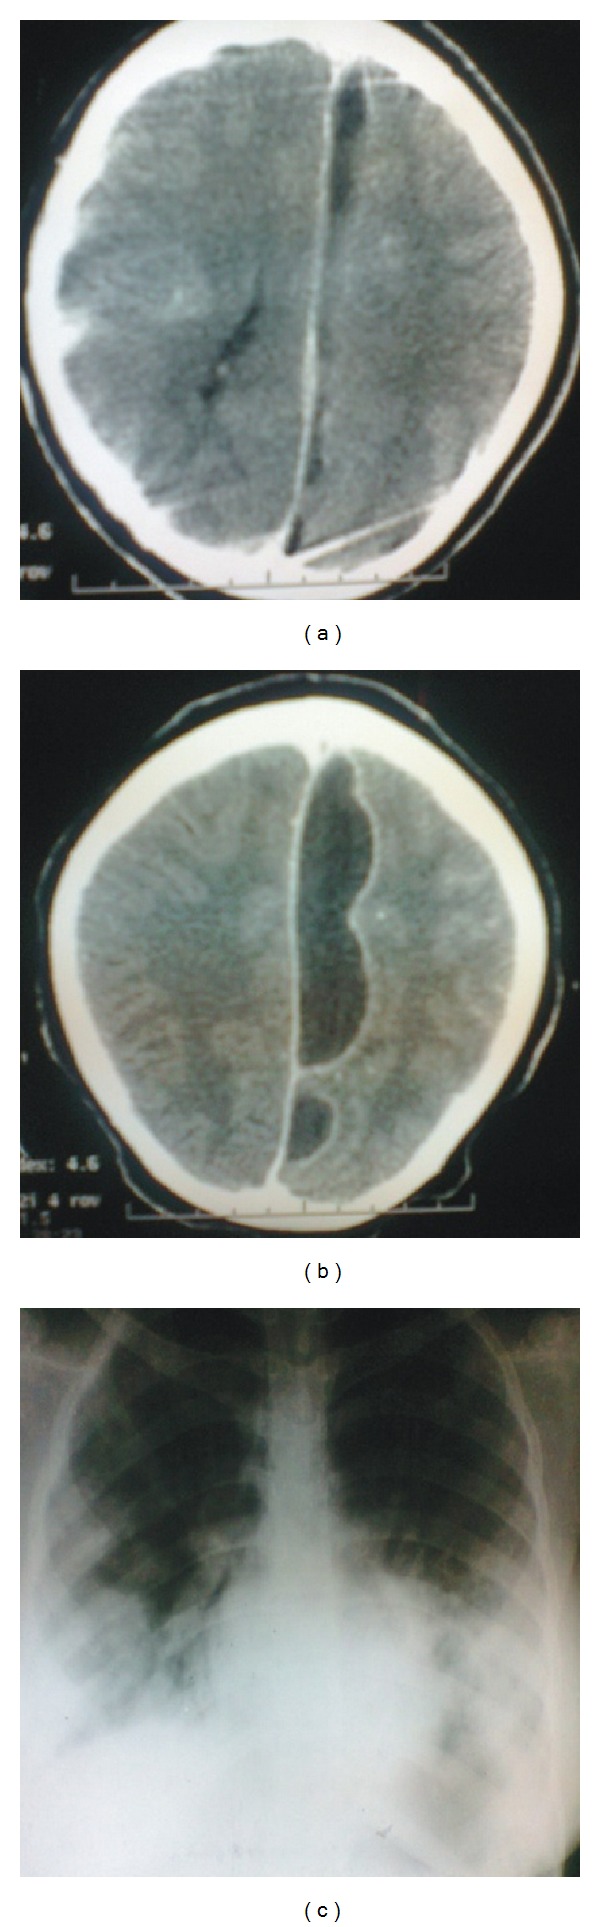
(a) Cranial CT scan of the patient showing a hypodense lesion in the parafalcine region with rim enhancement and effacement of the anterior of horn and body of the ipsilateral ventricle, consistent with superior sagittal sinus thrombosis or a left-parasagittal abscess; (b) a repeat scan 4 weeks later confirmed a marked progression of the lesion without significant shift of midline structures; and (c) CXR (AP view) shows bilateral mid and lower lobe opacities consistent with pneumonia.
